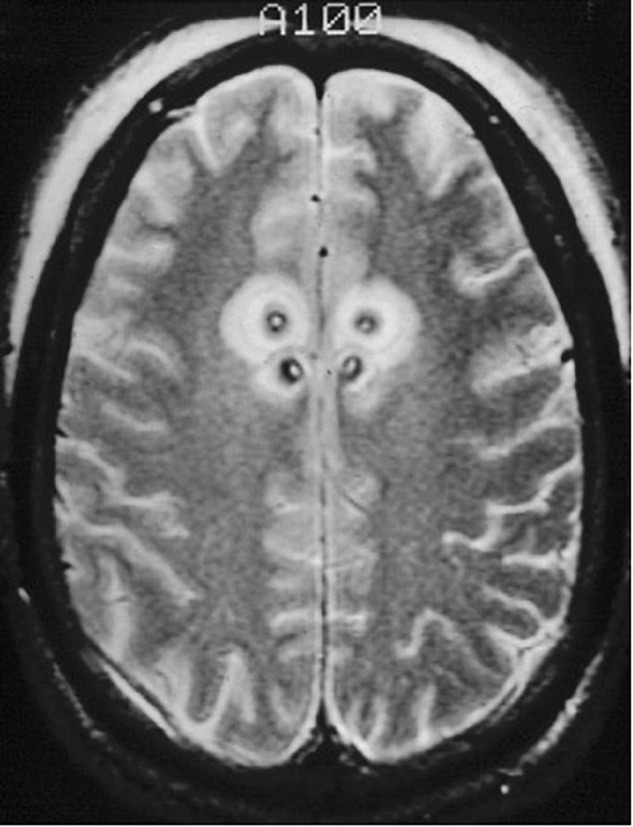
Staged cingulotomy. Transverse T2-weighted MRI of chronic (posterior) and acute (anterior) double cingulotomy lesions.
